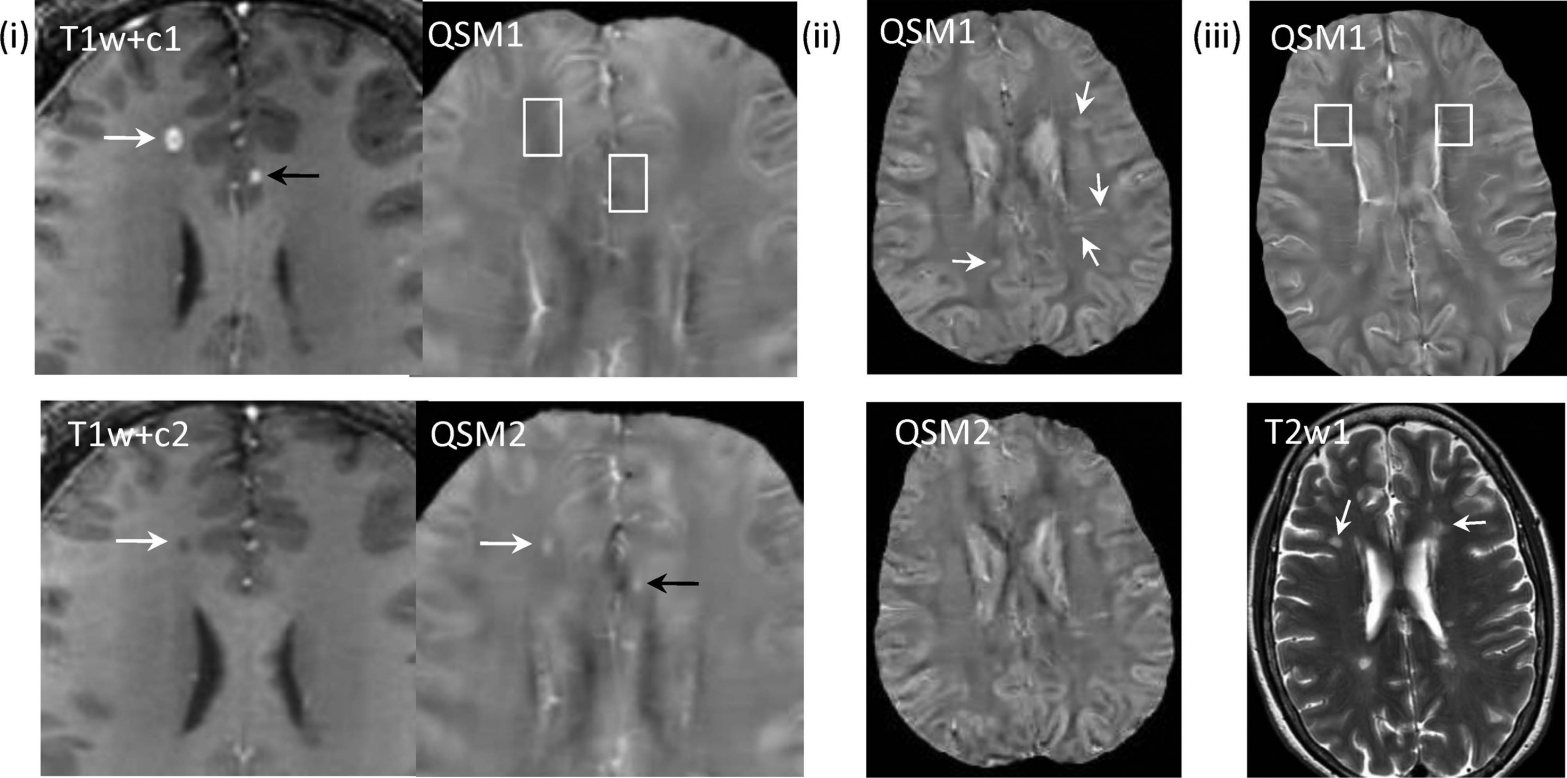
Quantitative susceptibility mapping for quantifying a mixture of paramagnetic and diamagnetic biomaterials. (i) Acute enhancing lesions in a 32-year-old male with RRMS at two time points: initial study (T1w + c1 and QSM1, 1st row) and 3-month follow-up (T1w + c2 and QSM2, 2nd row) (T1w + c – T1-weighted imaging with Gd). Lesions appear in the right frontal WM (white arrows) and in the lcc (black arrows). Both lesions are enhancing (arrows) on T1w + c1 and isointense (white boxes) on QSM1. The lesions changed on follow up to nonenhancing on T1w + c2 and hyperintense on QSM2 (arrows). The right frontal WM matter lesion shrunk between QSM1 and QSM2. The lcc lesion (black arrow) recovered to normal appearing on T2w and T1w (not shown), T1w + c. (ii) Nonenhancing lesions (33y, f, RRMS) on QSM at two time points (2nd row was 6 months later). All QSM lesions at time point 1 were estimated to be 1.2y using prior MRIs. All lesions (arrows) are QSM hyperintense on both time points with similar values. (iii) Chronic nonenhancing lesions (50y f RRMS) on QSM and T2W. Two lesions over 10 years old were detected (white arrows). They appear isointense on both QSM (white boxes, only initial study shown). lcc, left cingulate cortex; QSM, quantitative susceptibility mapping; RRMS, relapsing-remitting multiple sclerosis; WM, white matter. Source: Chen et al, Radiology 2014;271:183–192.
